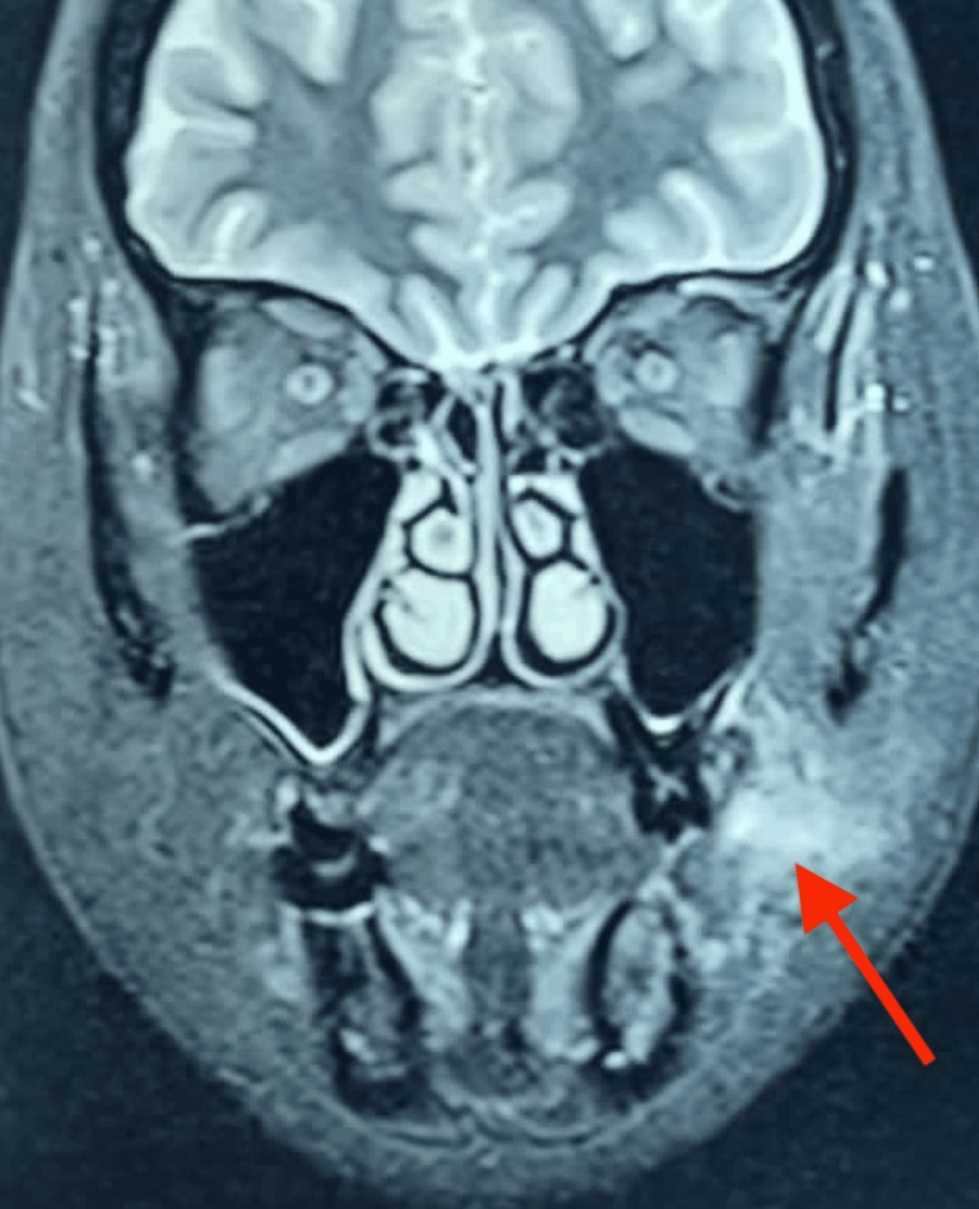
Coronal T2-weighted MRI sequence shows fluid-filled thick sinus on the left side
